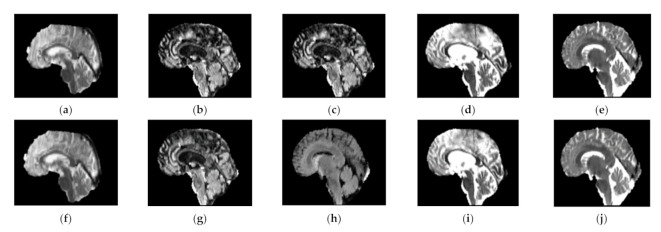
BraTS MR images, before (top row) and after (bottom row) Gibbs ringing artifact removal. Each MR image in top row (a–e) has the corresponding Gibbs ringing artifact-removed MR image (f–j) in the bottom row. All the images shown here are T1ce-saggital (for fair comparison). However, other sequences, i.e., FLAIR, T1 and T2 can also be addressed for ringing artifacts.
